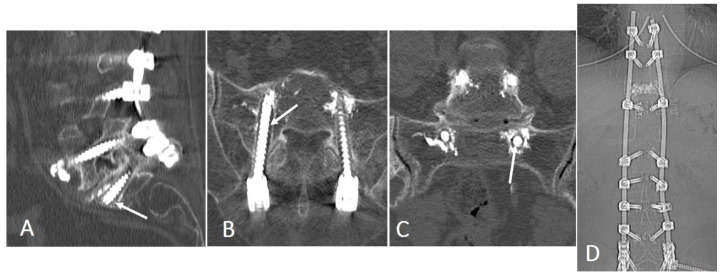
Postoperative CT images. (A–C) Axial and sagittal views showing bilateral S1 screw loosening with circumferential osteolysis of the surrounding bone (arrows). (D) Extended reconstruction demonstrating the implanted hardware in situ.
